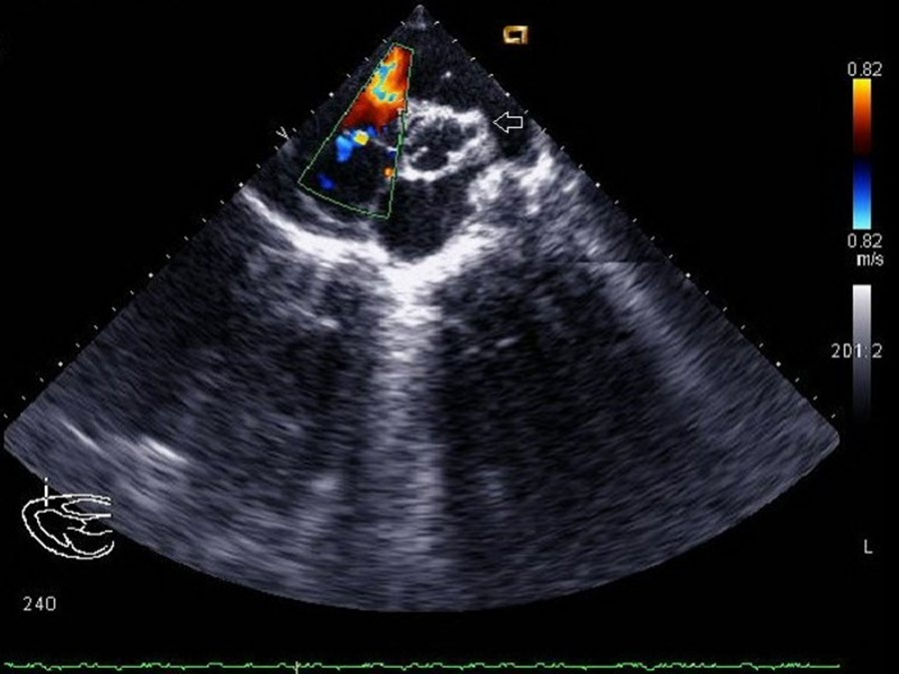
Color Doppler echocardiography of parasternal short axis view with leftward swept showing turbulent flow across the DCRV and mild tricuspid regurgitation along with left coronary artery aneurysm (*white arrowhead*)
